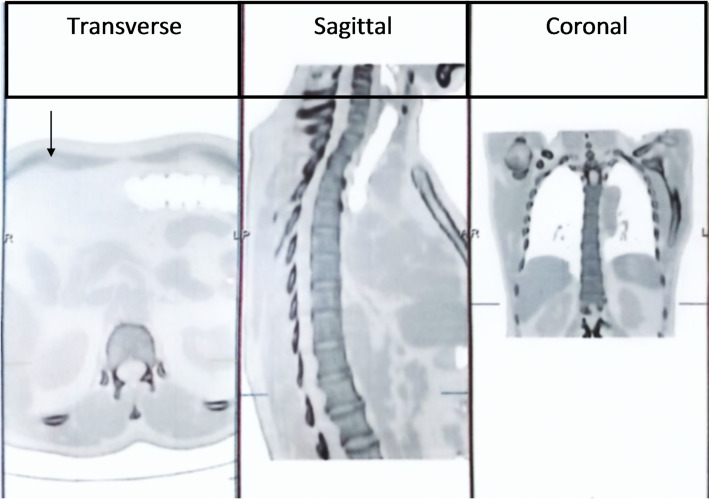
SPECT/CT Findings of Chest. A Transverse, B Sagittal, C Coronal slices of SPECT/CT images (arrow) of male breast cancer patient showing incidental lytic lesions in right rib
